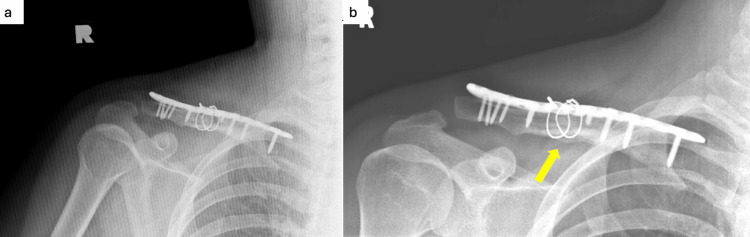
Plain radiograph of the right shoulder post revision surgery. (a) Immediate post-operative X-ray: distal clavicle locking plate inserted and cerclage wire used to hold olecranon cortical bone graft to the clavicle. (b) X-ray at four months post-op: callus formation (yellow arrow).
